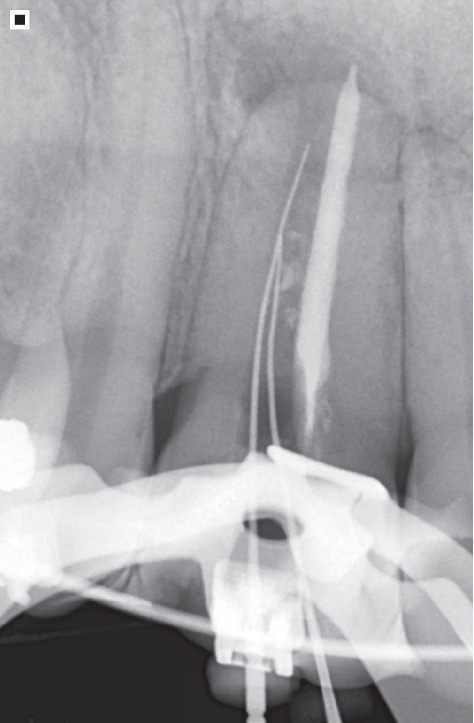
Periapical radiograph, where it can be observed using an endodontic file that there is an untreated root canal. Additionally, a radiolucent area is observed at the root apex.
